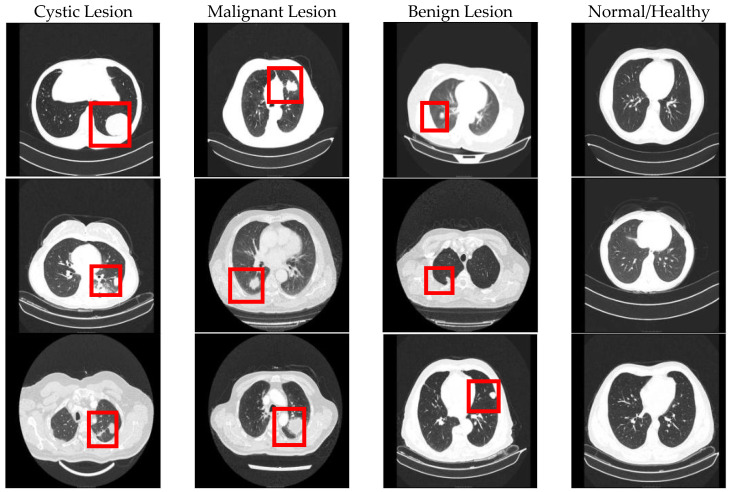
Example CT images in the datasets.
